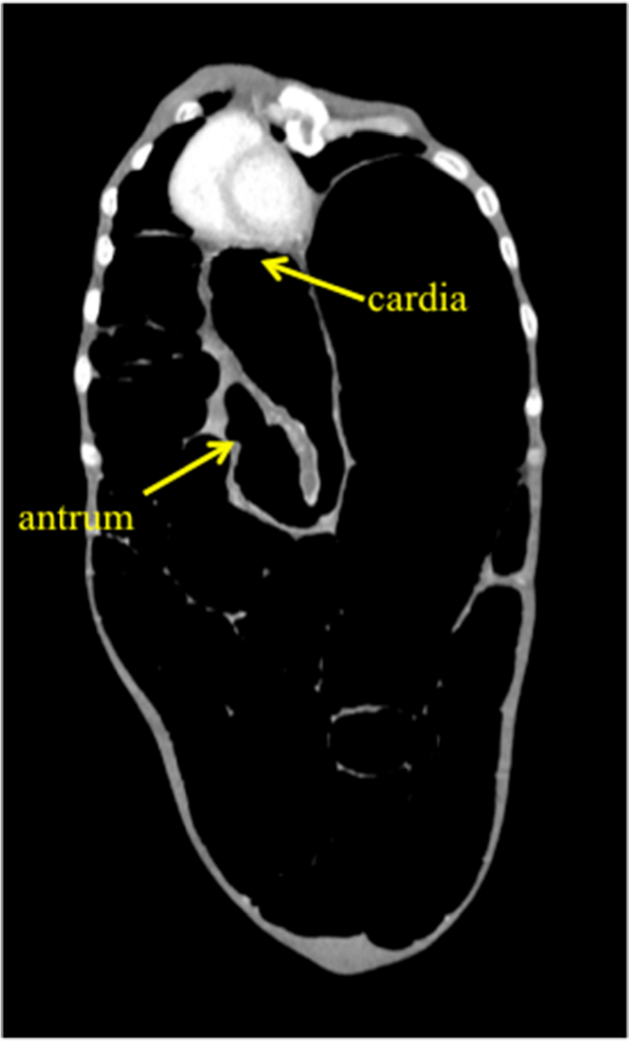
Postoperative findings. The coronal view of the enhanced CT revealed the recovery of gastric volvulus after surgery.
